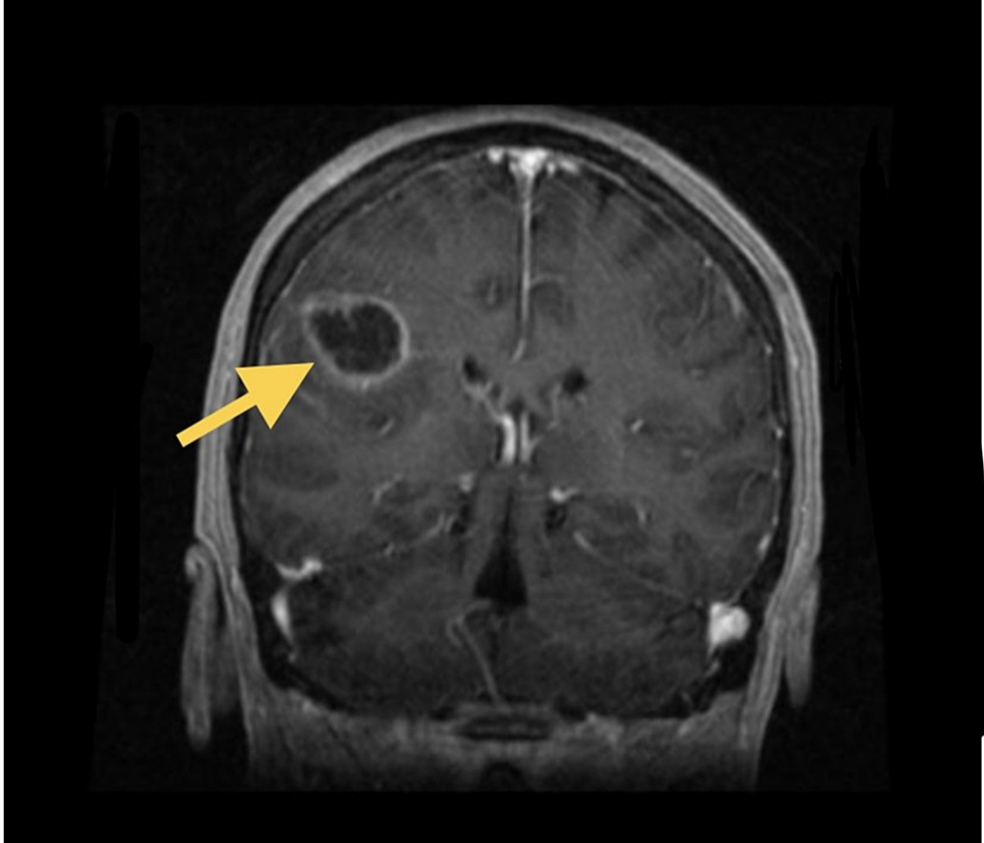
Coronal view on MRI flair with the yellow arrow showing posterior right frontal lobe mass.
